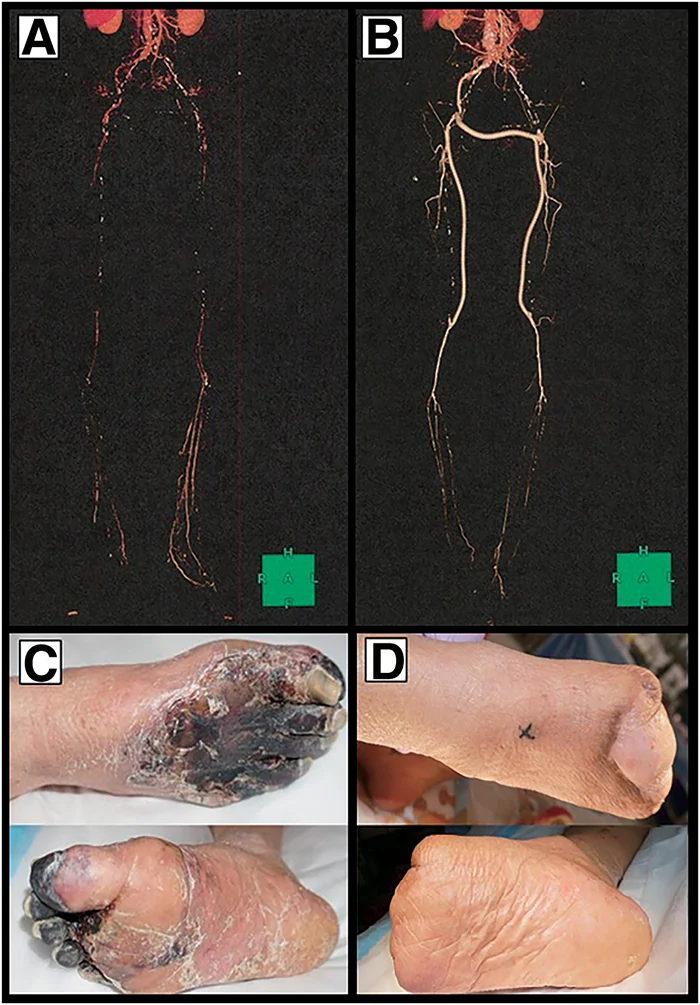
Preoperative and postoperative contrast-enhanced CT and foot findings. (A) Three-dimensional reconstructed images from preoperative contrast-enhanced CT. (B) Three-dimensional reconstructed images from postoperative contrast-enhanced CT. (C) Preoperative photograph of the right foot. (D) Photograph of the right foot, which had fully healed following revascularization surgery and metatarsectomy. CT, computed tomography
